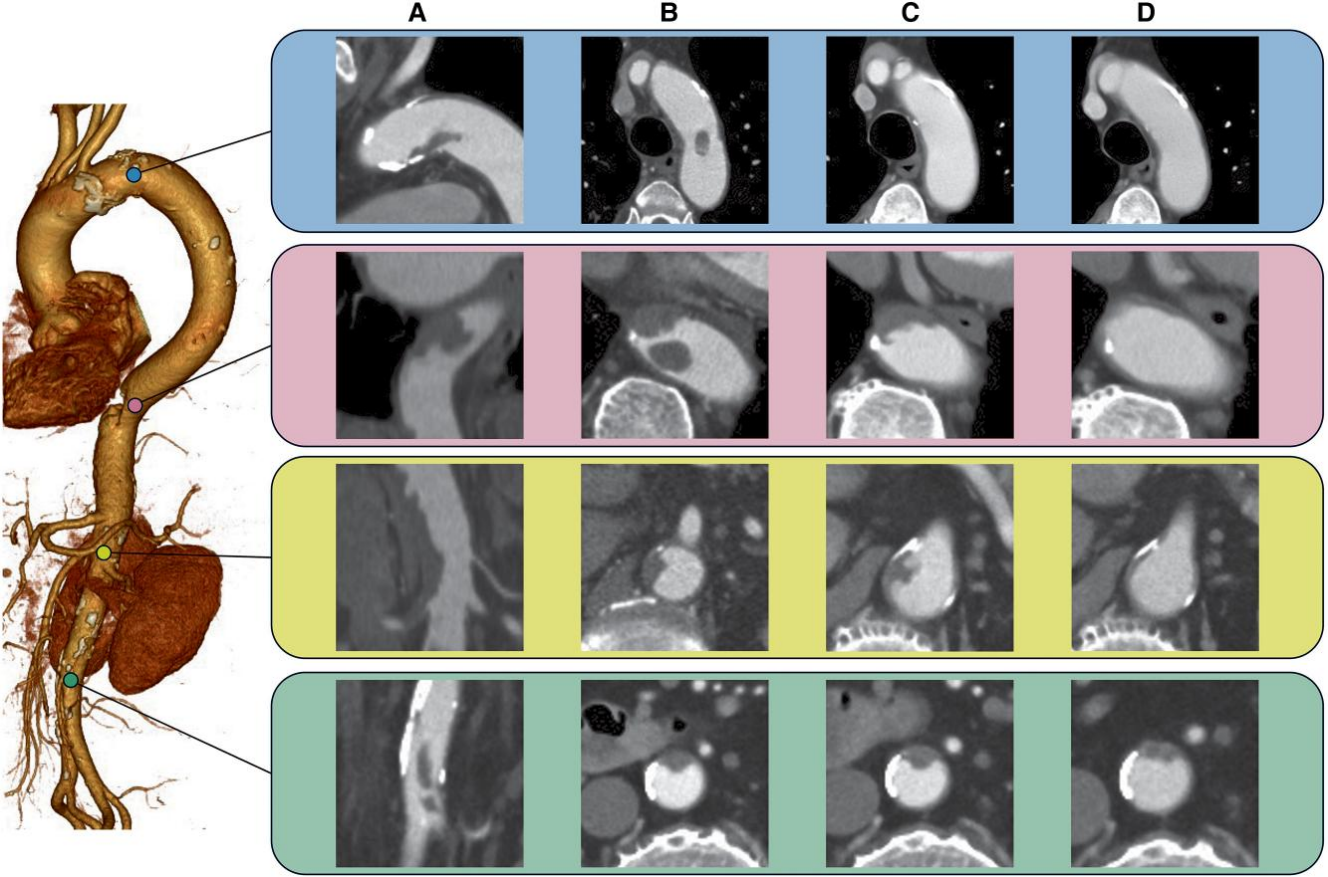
An 89-year-old man presented to the emergency department with abdominal pain. Computed tomography showed multiple aortic floating thrombi (MAFT) (*Panels A* and *B*) and transthoracic echo showed a floating thrombus in the descending aorta (see *Supplementary material online, Video S1*). Laboratory tests showed a platelet count of 120 × 10^4^/μL and bone marrow aspiration revealed primary myelofibrosis leading to abnormal thrombocytosis. Direct oral anticoagulants (DOACs) and antiplatelet agents were administered for MAFT and thrombocytosis. We followed the process of MAFT resolution for 4 weeks (*Panel C*) and 8 weeks (*Panel D*) without visceral branches and peripheral arterial emboli. MAFT is a rare disease that can be catastrophic due to visceral branches and peripheral arterial embolism.
